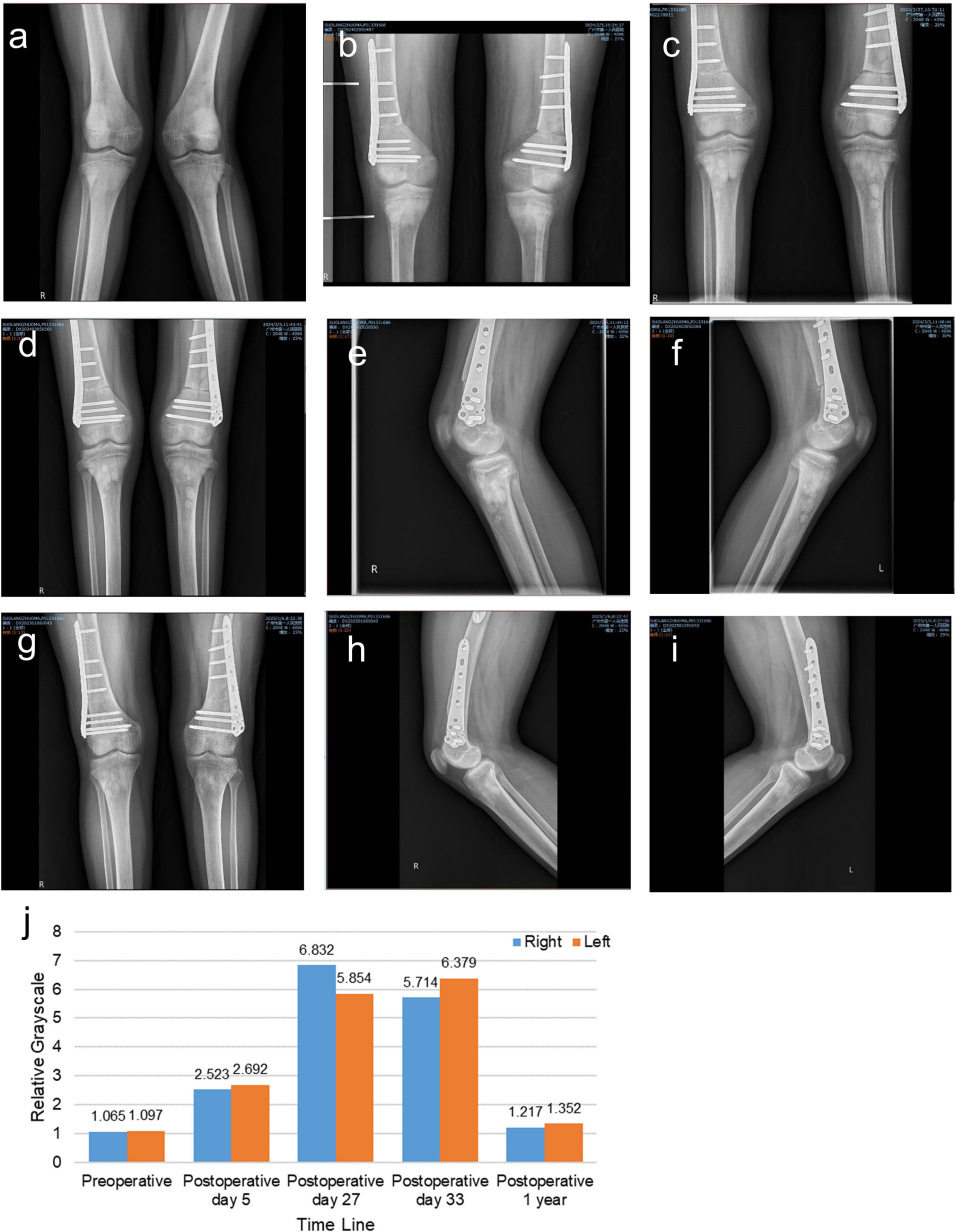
X-ray evaluation of transient osteosclerosis during the follow-up. (a) Preoperative radiograph showing normal bone density. (b) Diffuse periarticular hyperdensity (tibial metaphysis) on postoperative day 5. (c) Multifocal osteosclerotic regions (tibial metaphysis) emerged on postoperative day 27. (d–f) Stable lesions on postoperative day 33. (g–i) Near-complete resolution of hyperdensity at 1 year, with no pathological changes. (j) Quantitative analysis of transient osteosclerosis.​​ The analysis was performed using ImageJ software (National Institutes of Health, USA). Briefly, radiographs were imported, converted to 8-bit grayscale images, and two rectangular regions of interest (ROIs) of equal size were defined: one encompassing the proximal 10 cm of the tibia (including the metaphyseal sclerotic regions) and another covering the subsequent 10 cm of the tibial diaphysis distally. The relative grayscale value was calculated by dividing the mean pixel density of the proximal ROI by that of the distal ROI. The initial elevation and subsequent normalization of the relative grayscale value objectively demonstrate the appearance and resolution of the hyperdense sclerotic regions.
